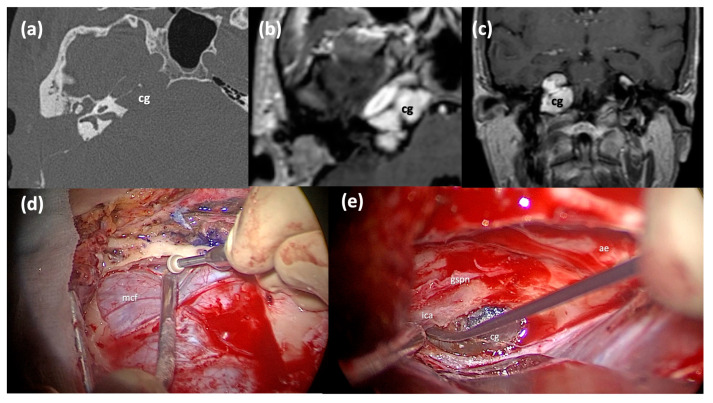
Middle cranial fossa approach. (a) Axial CT scan of a right Type C PACG with erosion of the horizontal ICA bone walls. (b,c) Axial and Coronal T1-weighted MRI sections of the same PACG, showing the typical hyperintense signal. (d) Surgical step: a craniotomy has been performed and the dura of the middle cranial fossa is carefully elevated from the skull base. (e) Surgical step: the lesion is identified after the Kawase triangle drilling. cg: cholesterol granuloma; mcf, middle fossa dura; gspn, greater superficial petrosal nerve; ae, arcuate eminence; ica, internal carotid artery.
